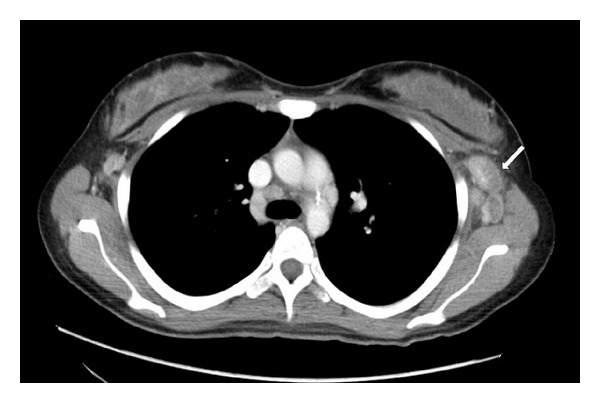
Axillary lymphadenopathy on CT thorax with contrast.
